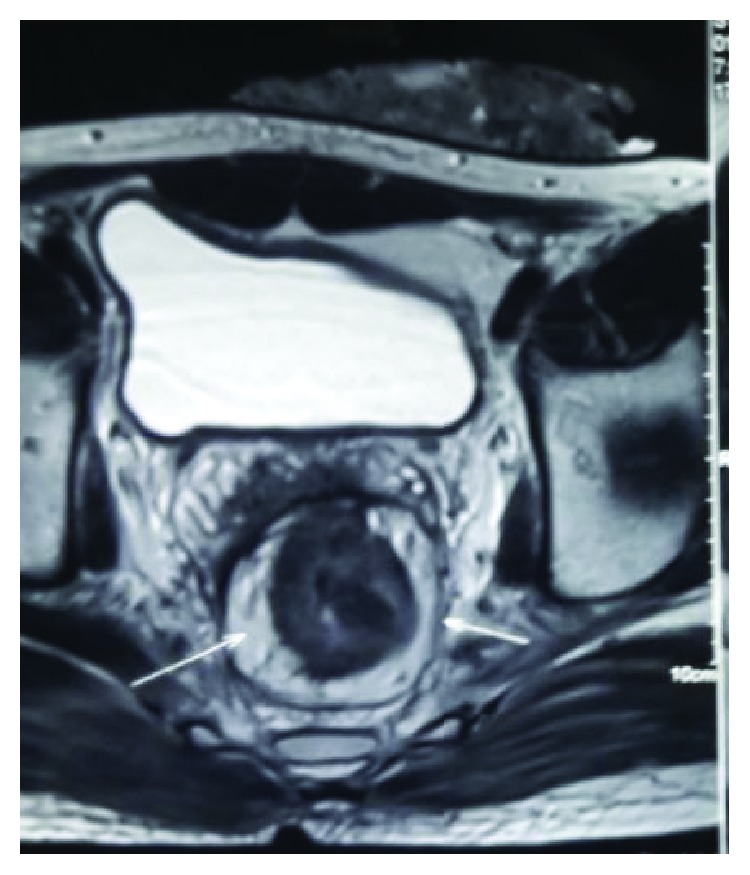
MRI pelvis of the same patient showing circumferential thickening of the rectum.
